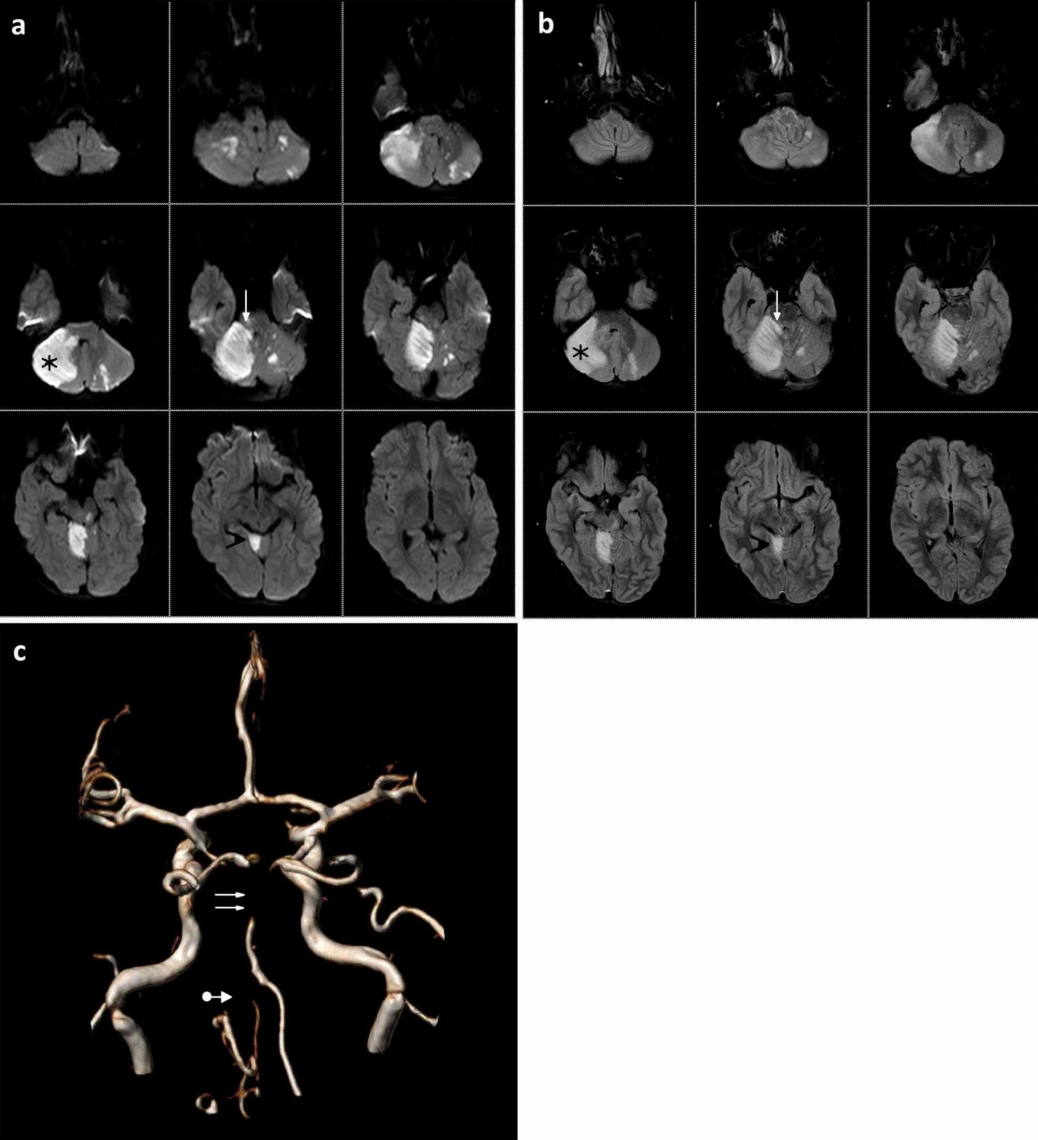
Admission magnetic resonance imaging (MRI) An MRI scan taken upon the patient’s arrival that demonstrates: a) a hyperintense hyperacute ischaemic area in the right cerebellar hemisphere (black star) and in the right-sided vermis (black arrowhead) with partial involvement of the right middle cerebellar peduncle and the upper cerebellar peduncle (white arrow) in diffusion-weighted imaging (DWI) sequence; b) a hyperintense acute ischaemic area in the right cerebellar hemisphere (black star) and in the right-sided vermis (black arrowhead) with partial involvement of the right middle cerebellar peduncle and the upper cerebellar peduncle (white arrow) in fluid-attenuated inversion recovery (FLAIR) sequence; c) magnetic resonance angiography (MRA) shows the absence of flow in the middle-distal tract of the basilar artery (double white arrows) with a reduction similar to that of both vertebral arteries (white arrow with dot)
